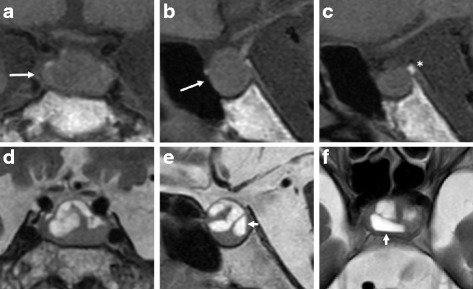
Magnetic resonance imaging of the brain (July 2016). Plane T1-weighted images (a: coronal plain, b: sagittal plain) showing a 2.5-cm pituitary tumor (*arrows*) and deformed hypophyseal stalk, with no compression on the optic chiasm. The physiological high-intensity signal (*) was found in the posterior pituitary gland (c: sagittal plain). T2-weighted images (d: coronal plain, e: sagittal plain, f: transverse plain) revealed a mixed pattern of solid and liquid components in the pituitary tumor, with fluid-fluid levels (*short arrows*)
